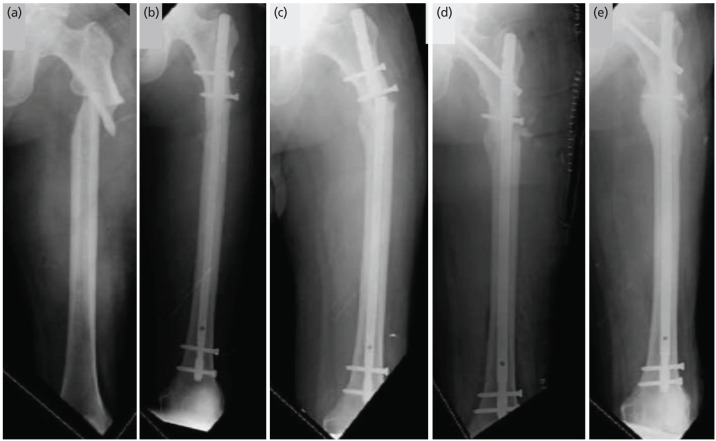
A total of 68-year male with H/O slip and fall with varus malreduction of 10.27°. (a) Pre-op radiographs, (b) post nailing radiograph, (c) implant failure with non-union at 21-month follow-up, (d) revision nailing with bone grafting, (e) united fracture at nine-month post-re-nailing and bone grafting.
